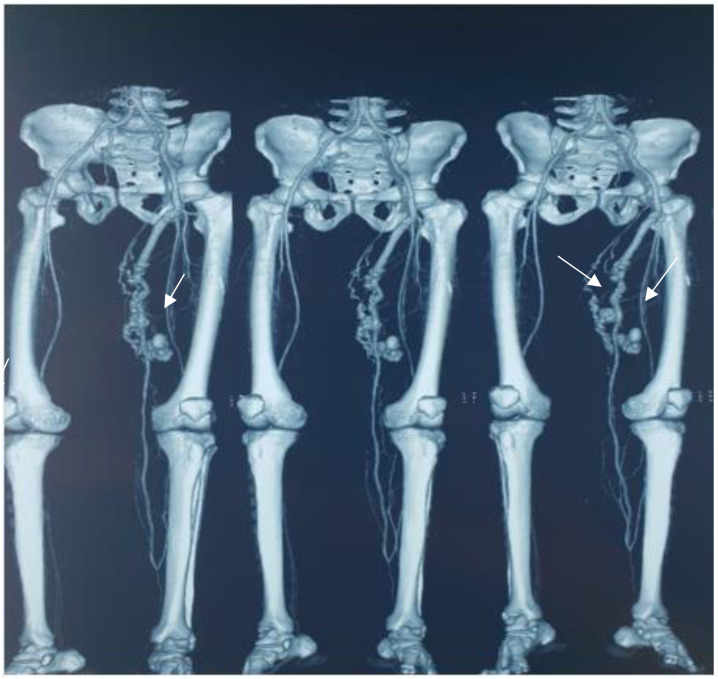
CT angiography of the lower limbs showing multiple arteriovenous fistulas complicating an in situ saphenous vein bypass. The femoral to posterior tibial artery bypass is a procedure used for revascularization of the lower limbs during critical limb ischemia. The conduit often used is the internal saphenous vein, which has a higher rate of patency than other vascular substitutes [1]. In situ saphenous vein bypass was first described by ROB [2]. The problem with this type of bypass is the persistence of a residual arteriovenous fistulas. These can cause localized edema, cellulitis, skin infarction, decreased distal flow and even graft thrombosis [3]. The rupture of an aneurysm of a residual arteriovenous fistula is an unusual late complication of an in situ saphenous graft motivated this report. A 48-year-old patient underwent a right femoral to posterior tibial artery bypass 5 years previously for critical lower limb ischemia. He was admitted to the emergency department for a ruptured varicose vein of the right lower limb with bright red blood. On examination, his blood pressure was 80/50 mm Hg, his heart rate was 110 cycles/minute and the presence of non-systematic varicose veins at the level of the lower third of the post face of the right thigh, surmounted by a small ulceration, was noted. In front of the antecedent of revascularization of the lower limb by a vein bypass, a CT angiography was done, which showed a patent bypass with multiple aneurysmal arteriovenous fistulas (Figure 1). The patient was operated on urgently. The surgical exploration confirmed the presence of multiple aneurysmal arteriovenous fistulas, which were excluded by section ligation (Figure 2). The postoperative course was simple.
